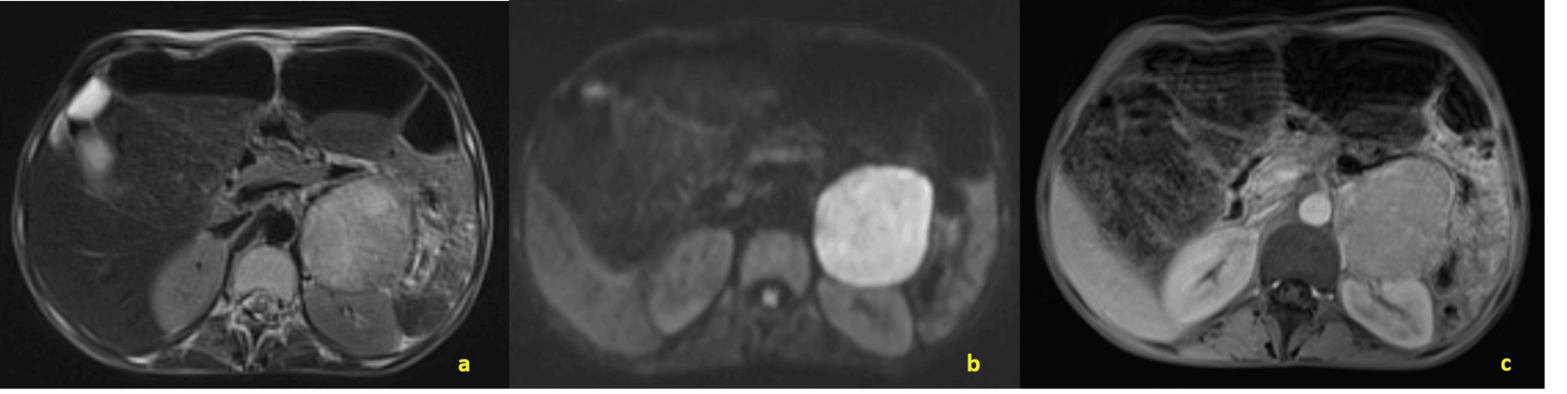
Abdominal MRI (axial views) of the left adrenal mass, including T2-weighted, diffusion-weighted, and post-gadolinium T1 sequences, showing heterogeneous hyperintensity on T2 and diffusion images and heterogeneous enhancement after gadolinium administration a: Axial T2-weighted image showing a well-circumscribed left adrenal mass with heterogeneous hyperintensity
